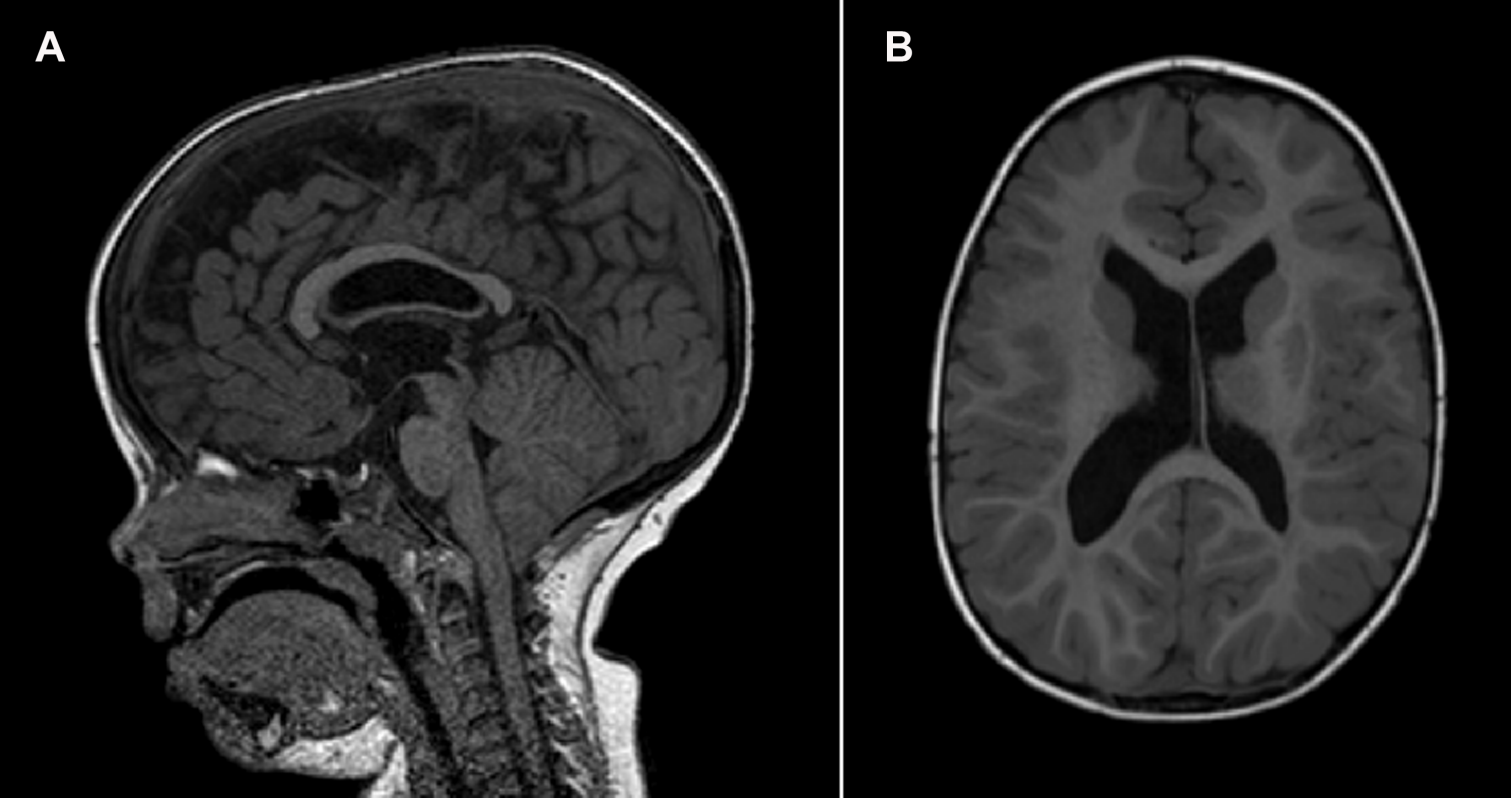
Brain MRI of patient 1 at 2.5 years of age. (A) Mid-sagittal T1-weighted image showing Chiari I malformation. (B) Axial T1-weighted image showing subependymal heterotopia.
